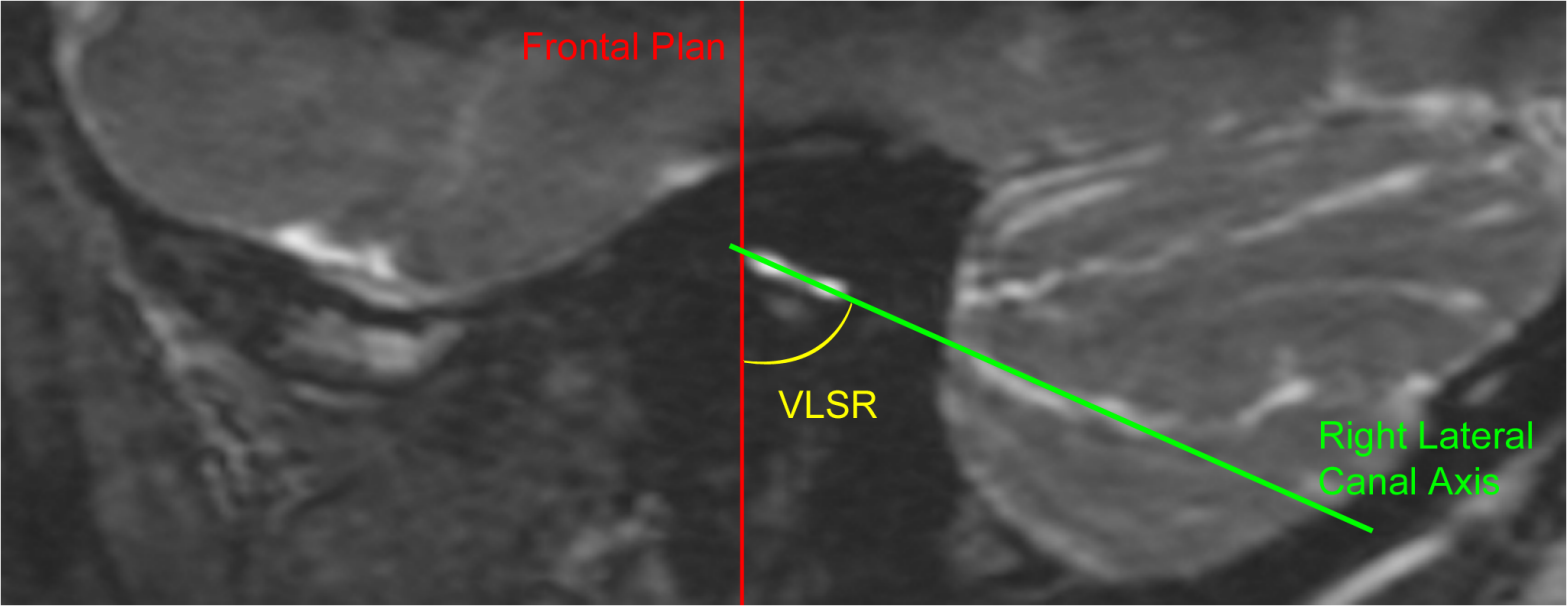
Measurement of lateral semicircular canal orientation in the parasagittal plane. T2 MRI image in the parasagittal plane showing the “
